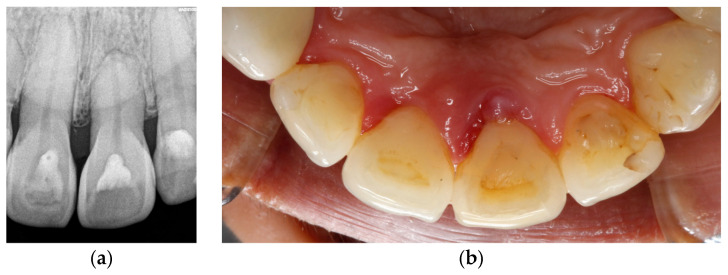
(a) Periapical radiograph of maxillary central incisor (2.1) showing root resorption. (b) Palatal restoration, edema, and traumatic occlusion.
